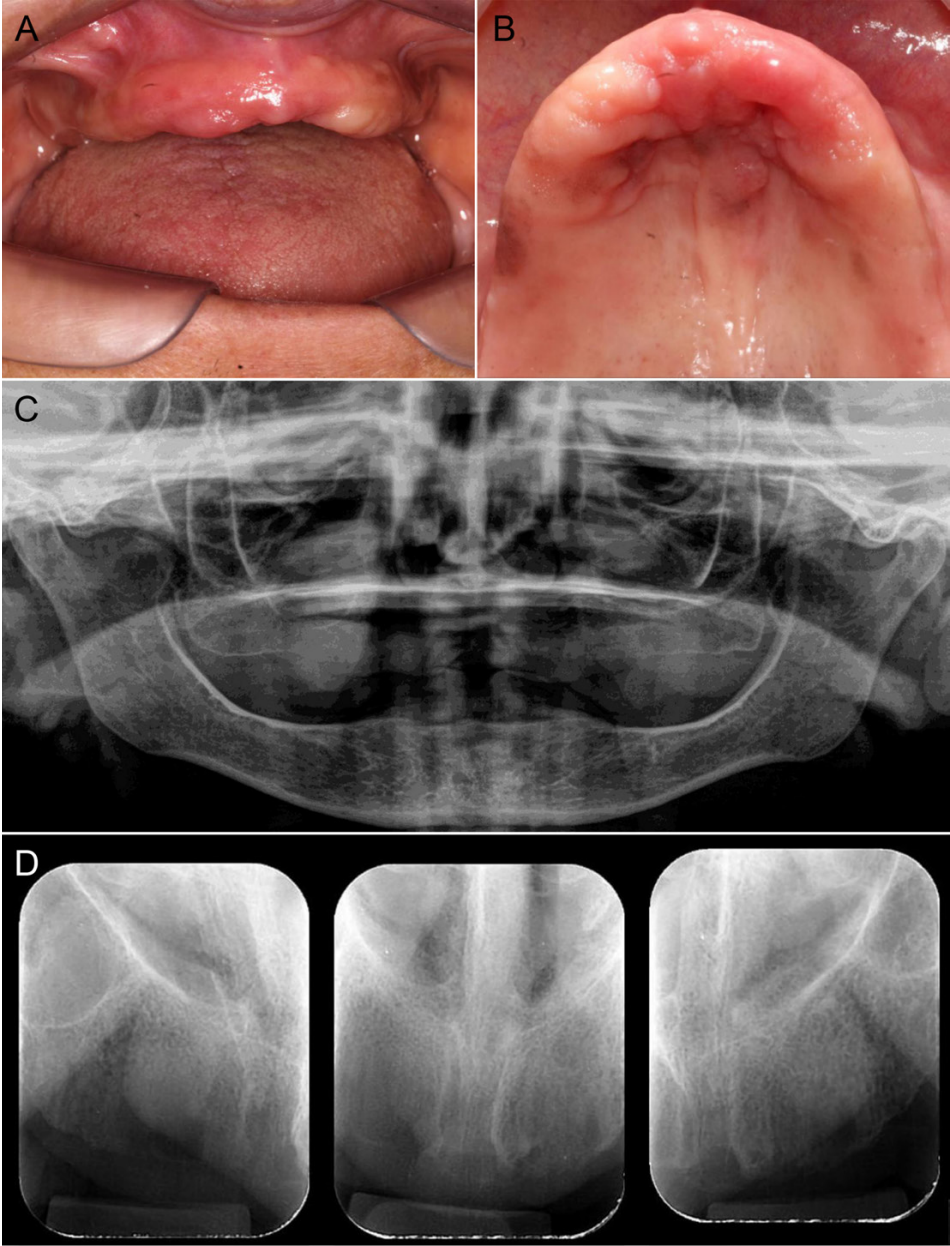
Clinical and radiographic features of oral amelanotic melanoma. (A) Intraoral view showing a completely edentulous maxilla. (B) Occlusal view of the maxilla revealing a single sessile, dome-shaped lesion located on the left transverse palatal rugae, measuring approximately 15×15×8 mm. The lesion exhibits a smooth surface and coloration similar to the adjacent mucosa. Discrete erythematous areas are observed on the anterior alveolar ridge, while the posterior and lateral ridges display multiple diffuse brownish macules of varying sizes. (C) Panoramic and (D) periapical radiographs showing no evidence of bone alterations.
